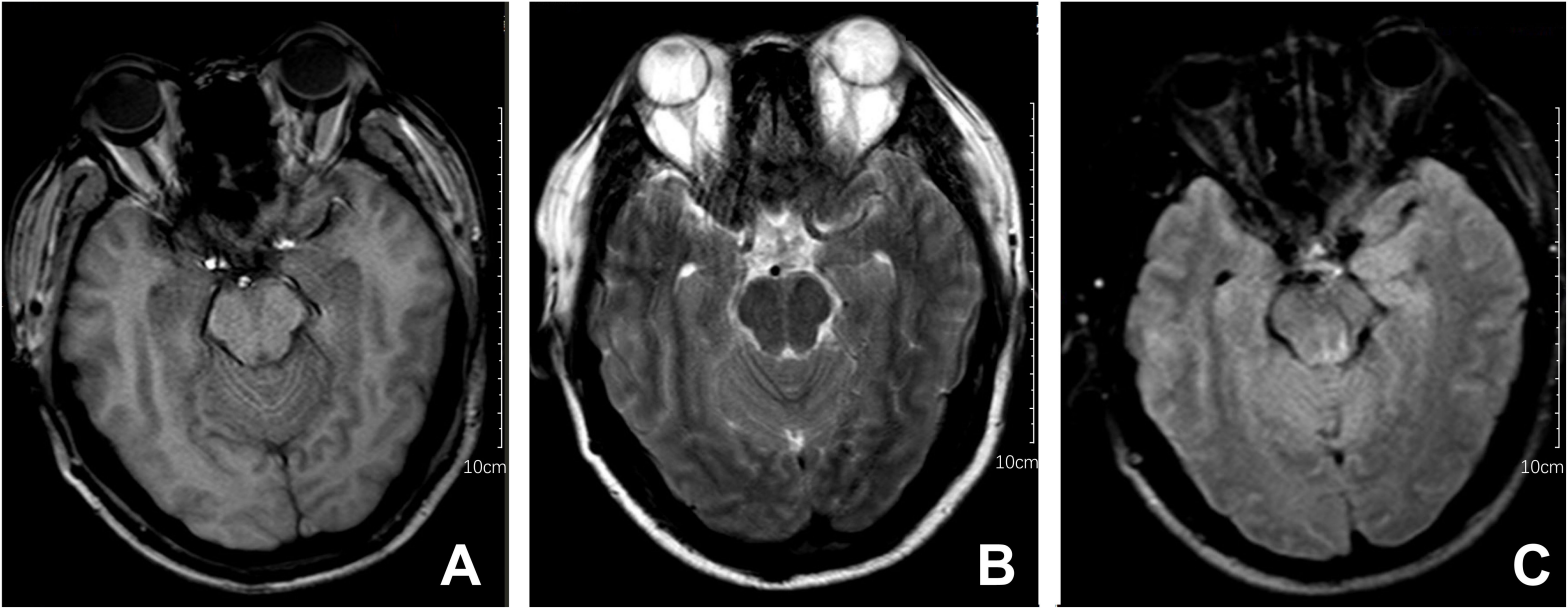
Brain MRI series of the patient. The following findings are described in the figure panel: (A) No abnormal signal is found on the T1 sequence; (B) Brain MRI revealed T2 hyperintense lesions in the cerebral cortex and bilateral hippocampus; (C) A corresponding fluid-attenuated inversion recovery (FLAIR) sequence shows extensive hyperintense signals in the cerebral cortex and bilateral hippocampus.
